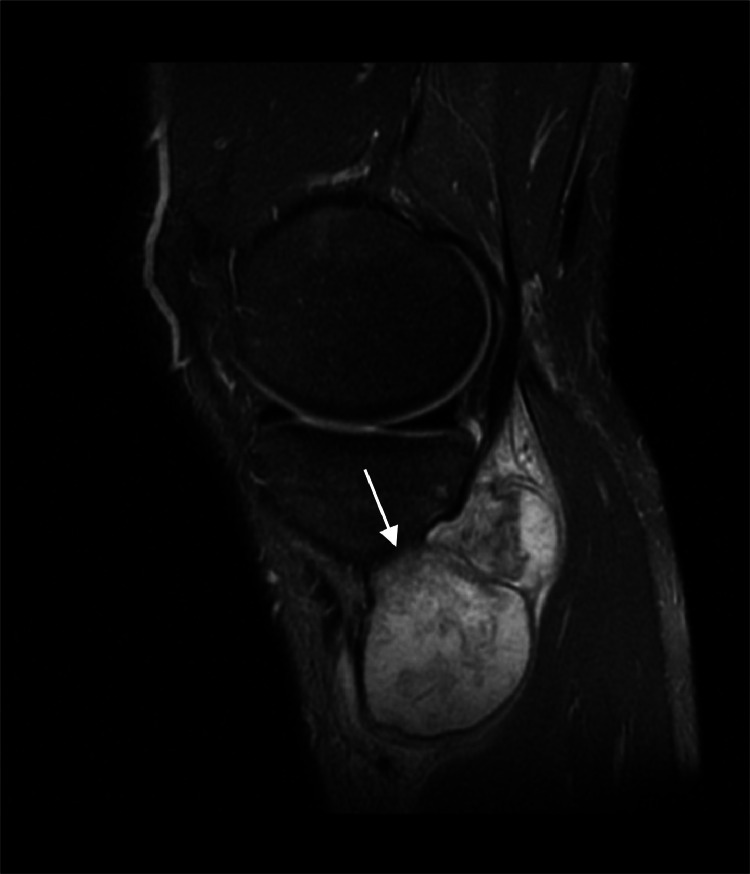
MRI of the left knee. Sagittal T2-weighted image shows a soft tissue mass (arrow) with heterogeneous hyperintense signal contiguous with the cortex of the proximal posteromedial tibial metaphysis.
